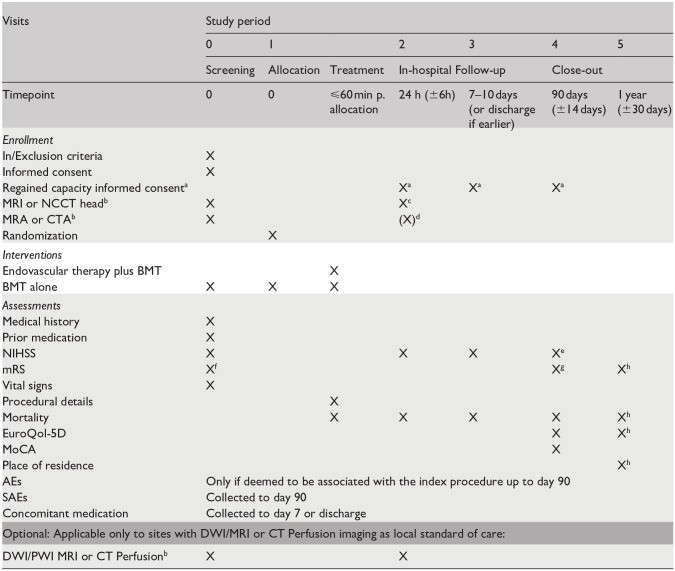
AE: adverse event; DWI: diffusion weighted imaging; NIHSS: National Institute of Health Score Scale; MoCA: Montreal Cognitive Assessment; mRS: modified Rankin Scale; MRI: magnet resonance imaging; NCCT: non contrast computed tomography; PWI: perfusion weighted imaging; SAE: serious adverse event.
